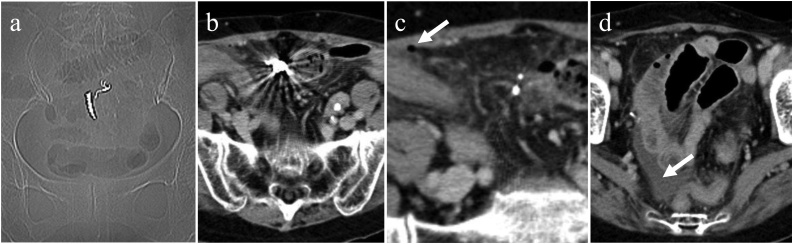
Findings from the radiograph and computed tomography (CT) scan of the abdomen in Case 2. (a) Radiograph showing a denture in the lower abdomen. (b) The denture was imaged as a hyperdense foreign body (FB) in the CT scan. (c) Abdominal free air (arrow) near the FB. (d) Ascites in the pelvic cavity (arrow).
